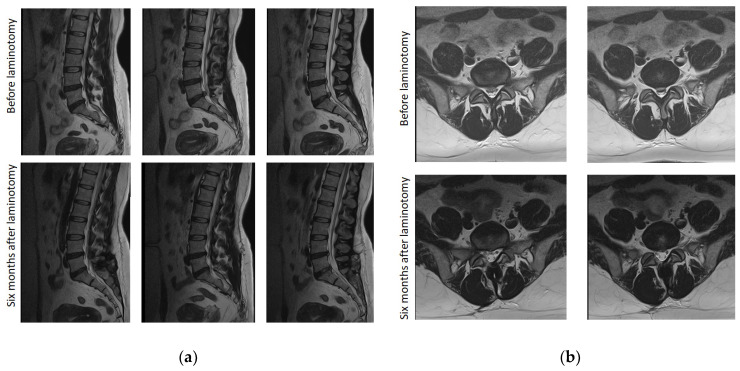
Sequential sagittal T2-weighted (a) and sequential axial T2-weighted (b) MRI of the lumbosacral spine with the development of severe spinal canal stenosis at the L5–S1 level, exceeding 60% before laminotomy, with complete resolution of the stenosis after surgery treatment in a 55-year-old woman. Despite good postoperative results, pain persists up to 9 points on VAS with a lack of tactile sensation up to 3 points in the L5, S1 dermatomes on the left leg and up to 3.5 points on the right leg. At the same time, the step activity averaged 2950 steps per day, symptom severity and functional disability of claudication according to the Zurich claudication questionnaire were 2.0 and 1.8 points, respectively, with a marked decrease in quality of life of up to 69%.
